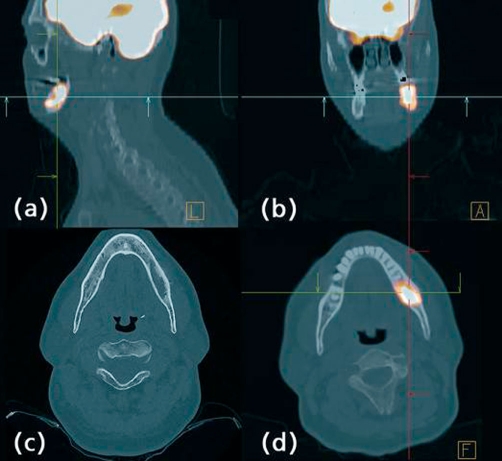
^18^FDG PET/CT showing the left mandible lesion in sagital (a), coronal (b) and axial (d) view. Image (c) shows an osteolytic lesion in the left mandible in an axial view of the CT scan.
